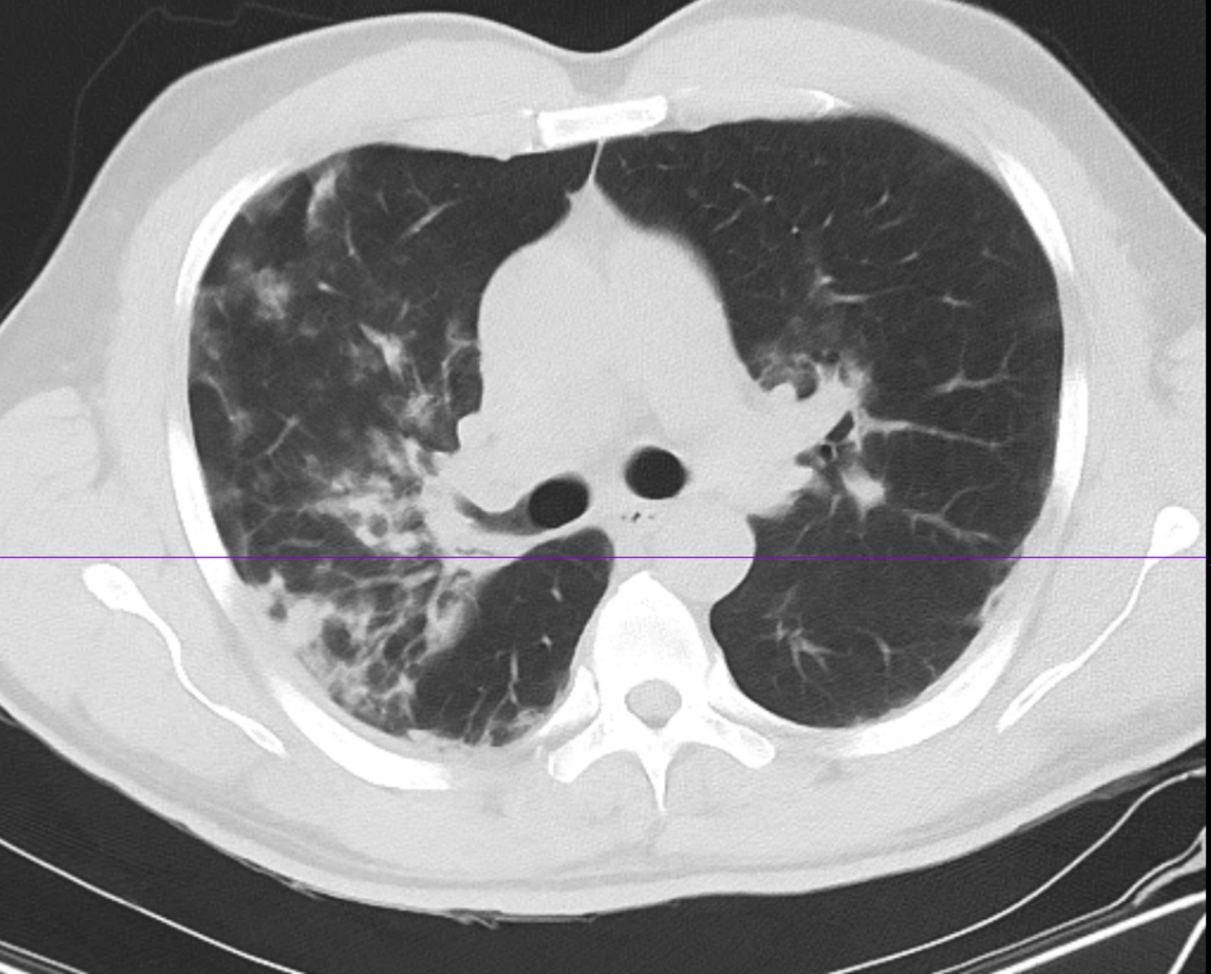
Case 3 chest CT on March 27, 2020 CT: computed tomography
